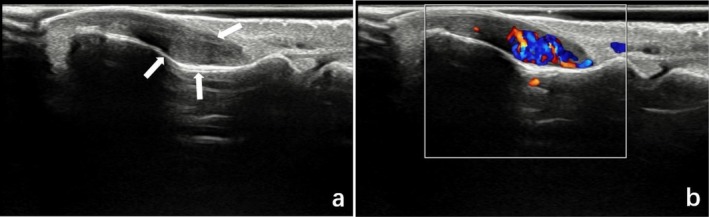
Subungual glomus tumor in a 37‐year‐old male (right thumb). (a) Grayscale ultrasound image demonstrates a well‐defined, ovoid hypoechoic mass (→) beneath the nail plate with posterior acoustic enhancement. (b) Color Doppler image reveals intralesional hypervascularity.
